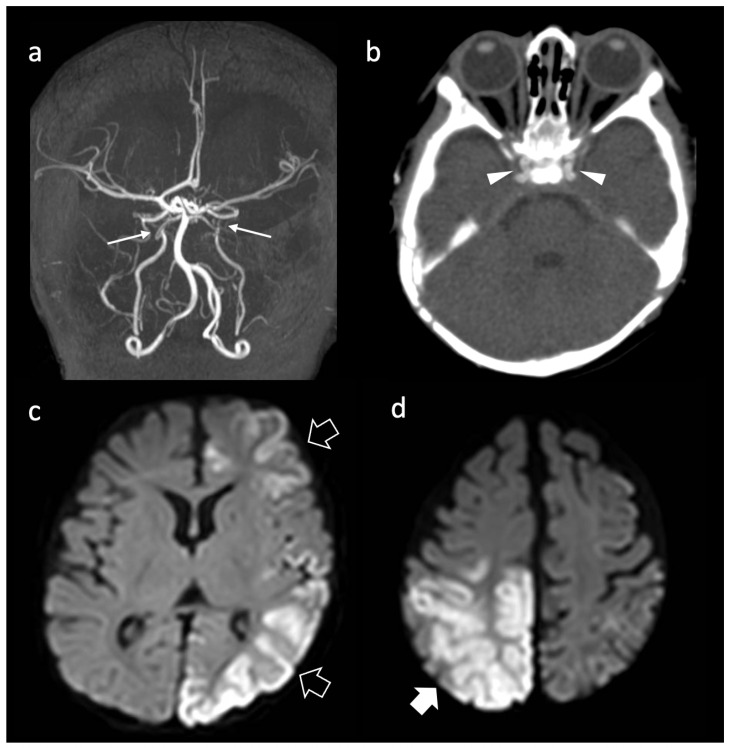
Neuroimaging features of patient 16 with GACI phenotype. (a) MRA, frontal view, reveals bilateral stenosis of the internal carotid arteries (thin arrows) associated with small collaterals. (b) Axial CT image demonstrates extensive calcifications of the affected vessels (arrowheads). (c,d) Axial diffusion-weighted images performed at clinical onset at 4 months of age (c) and 15 days later (d) demonstrate an arterial infarct in the left cerebral hemisphere (empty arrows) followed by a second arterial infarct in the right posterior frontal and parietal regions (thick arrow).
